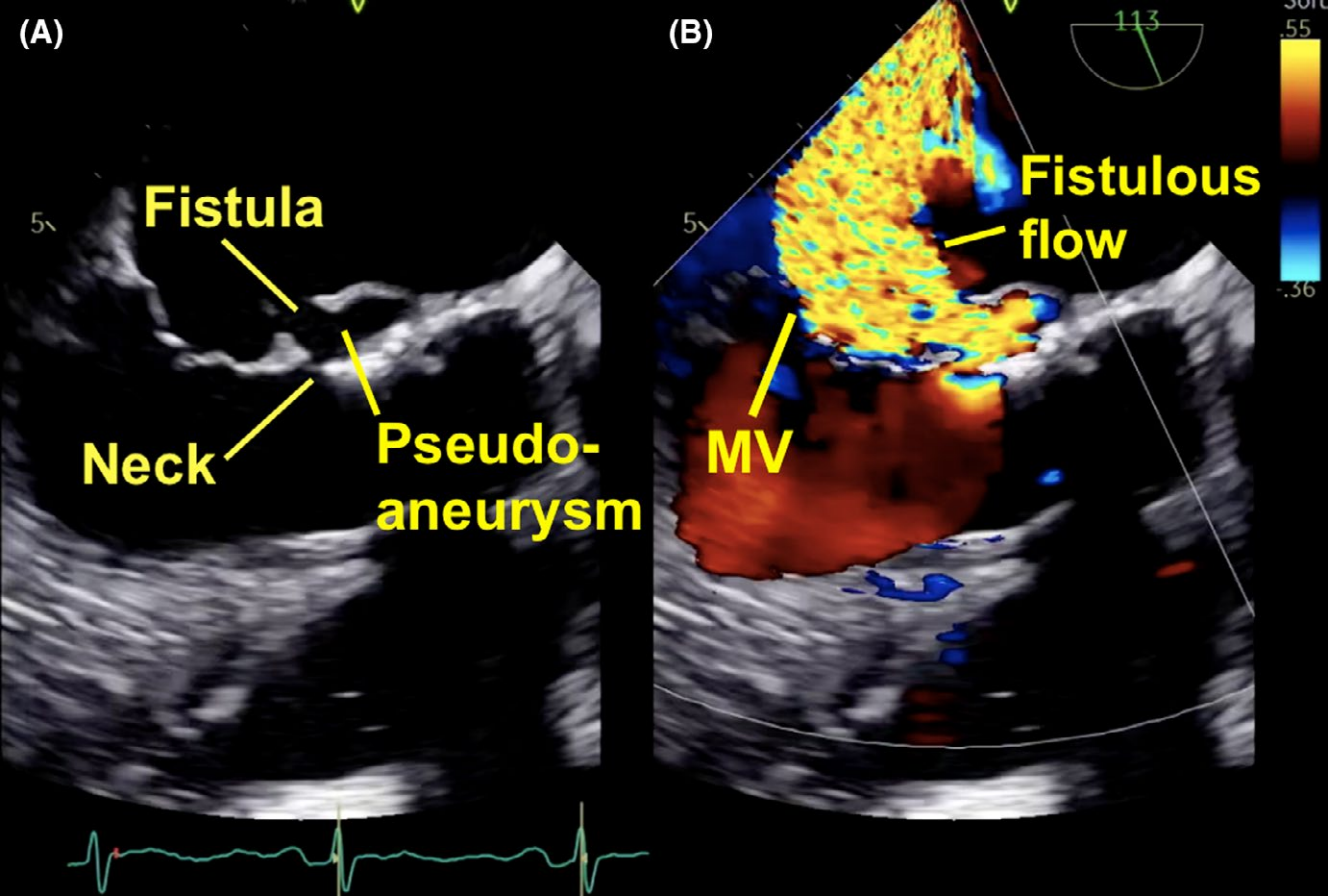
A, Mid‐esophageal transesophageal image revealing a perforated pseudoaneurysm of the mitral‐aortic intervalvular fibrosa. The perforation resulted in fistulous communication between the left ventricle and the left atrium. B, Color flow Doppler shows a large eccentric jet within the atrium coursing above the mitral valve (MV)
